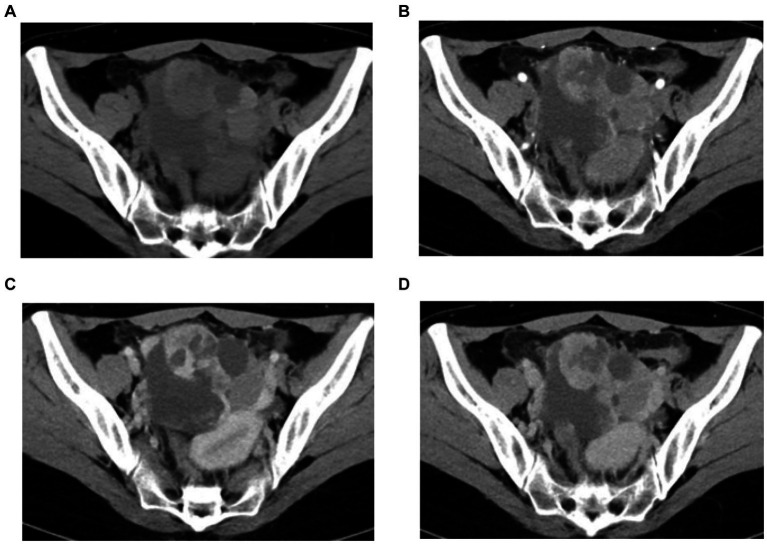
(A–D) Pelvic CT images. CT plain scan showed a left pelvic mass (A), contrast-enhanced CT showed a cystic solid pelvic mass with heterogeneous enhancement in the peripheral areas and a non-enhanced central area in arterial phas (B), venous phase (C), and delayed phase (D).
